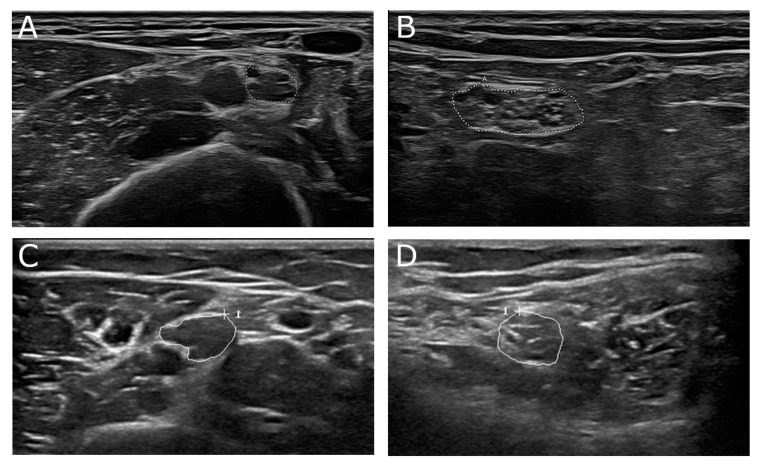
(A,B) 68-year-old male patient with genetically proven adrenomyeloneuropathy, an adult form of adrenoleukodystrophy. Nerve conduction studies showed a demyelinating damage pattern. In nerve ultrasound focal nerve enlargement was detected with a UPSS of 7 points. (A) Median nerve at upper arm segment (cross-sectional area (CSA) 15 mm^2^, normal value 12 mm^2^). (B) Tibial nerve at popliteal fossa (CSA 51 mm^2^, normal value 33 mm^2^). (C,D) 16-year-old female patient with juvenile form of metachromatic leukodystrophy. Focal nerve enlargement was seen in median nerve (C, CSA 20 mm^2^) at proximal nerve sites next to normal nerve segments in other nerves, i.e., tibial nerve (D, CSA 23 mm^2^). Areae enclosed by the discontinuous points in picture A and B depict CSA of the described nerve section, “A”means “area”. Areae enclosed by circles in pictures C and D depict CSA of the described nerve section, “+” shows curser position, “1” the count of measured areae.
